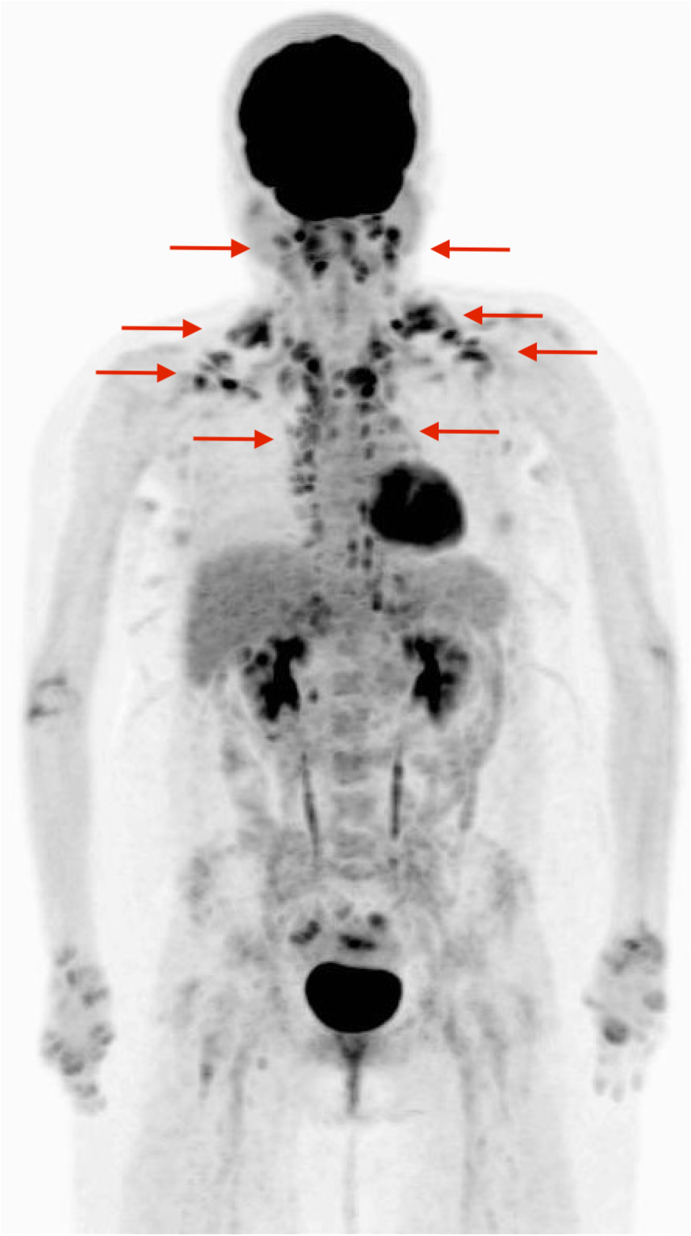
Representative 2-fluoro-2-deoxy-D-glucose positron emission tomography-computed tomography (FDG–PET–CT) image of active brown adipose tissue (BAT). Maximum-intensity projection positron emission tomography-computed tomography (PET–CT) image demonstrating increased [^18^F]2-fluoro-2-deoxy-D-glucose uptake in characteristic BAT deposits (red arrows) in the cervical, supraclavicular, and paravertebral regions.
